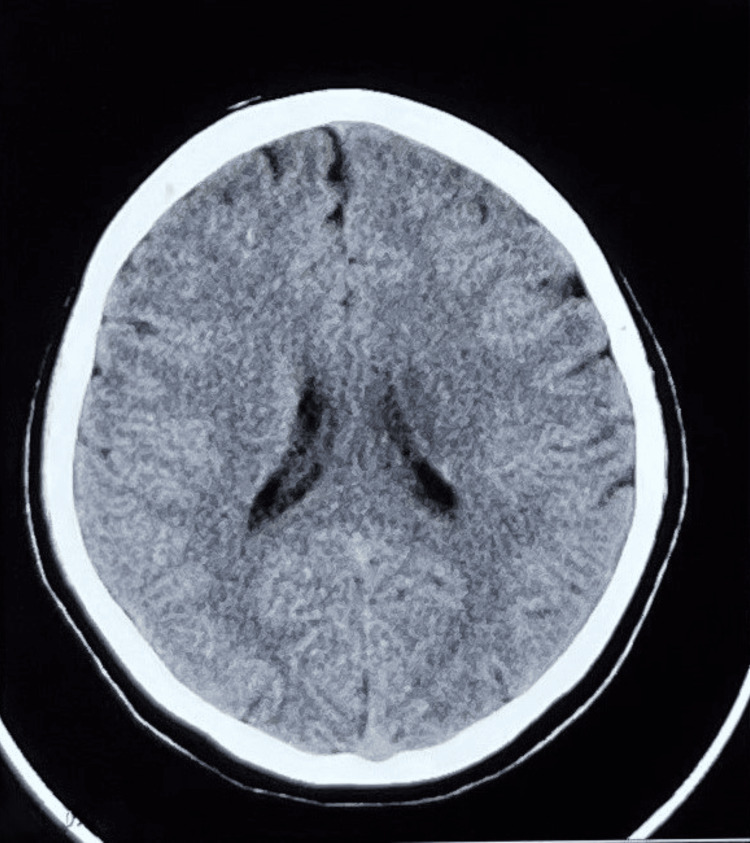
Non-contrast CT of the brain. Non-contrast CT of the brain is the first step to rule out hemorrhage in patients presenting with acute-onset focal neurological deficits. The CT revealed no evidence of hemorrhage and, hence, the focal neurological deficit was due to an ischemic phenomenon.
